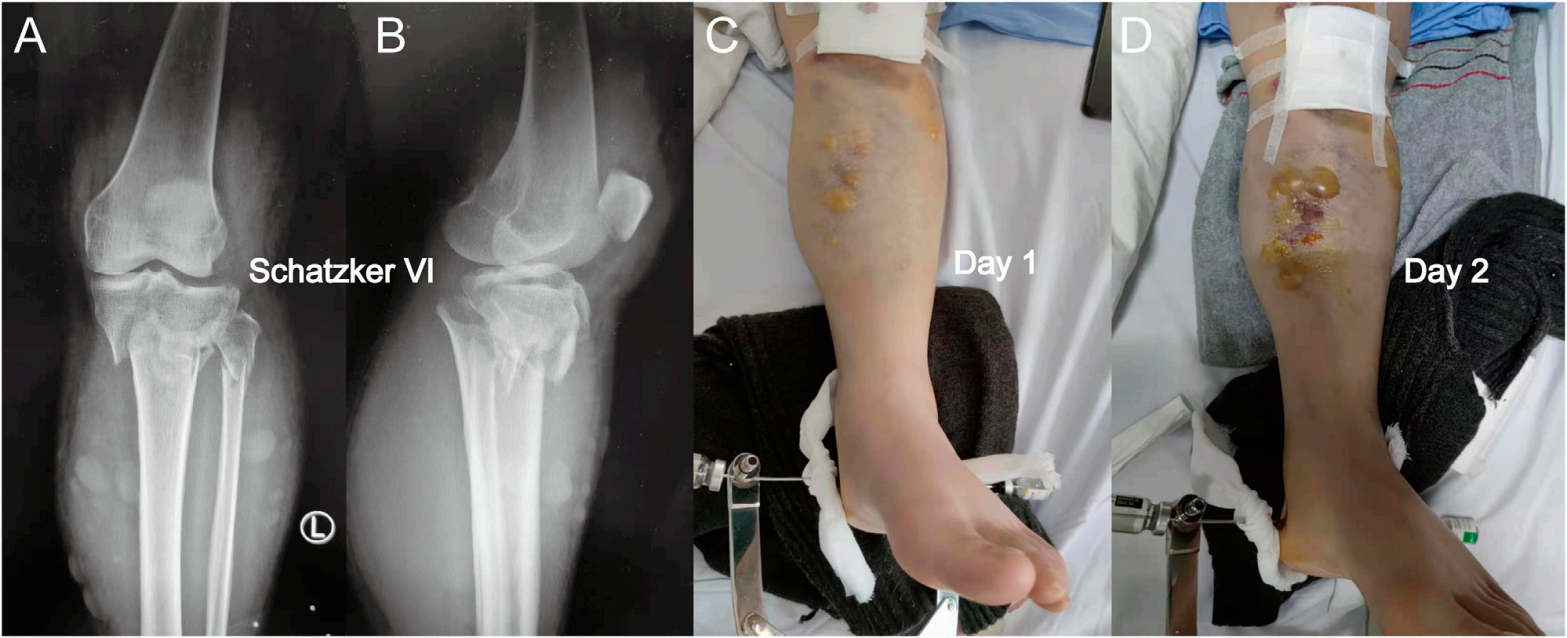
(A) Anterior-posterior X-rays of a Schatzker VI tibial plateau fracture. (B) Lateral view of this fracture. (C) Fracture appearance with a serous-filled blister observed on the skin around the fracture site (proximal tibia) after admission. (D) Blister became larger after 1 day, and the patients were not subjected to fasciotomy. When a blister was observed, the intracompartmental pressure decreased significantly.
